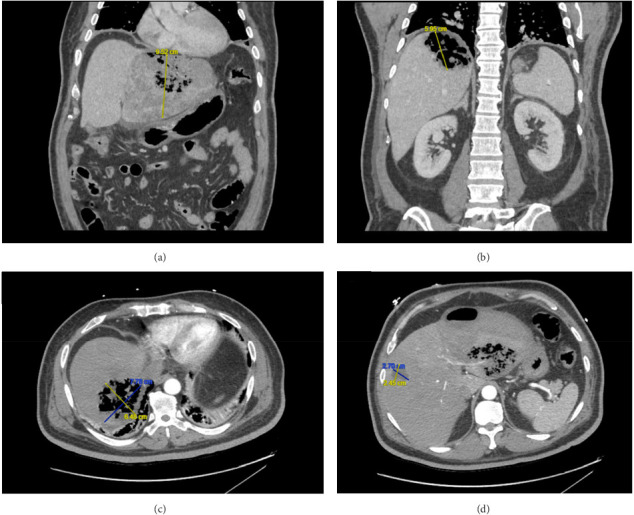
CT of the abdomen with IV contrast. (a) Coronal view of gas occupying the left hepatic lobe measuring 14.4 × 10.2 × 9.5 cm. (b) Coronal view of an additional similar collection within the right hepatic lobe measuring 7.8 × 6.5 × 5.95 cm. (c) Axial view of an additional similar collection within the right hepatic lobe measuring 7.8 × 6.5 × 5.95 cm. (d) Axial view of rim-enhancing collection in the right hepatic lobe measuring 2.8 × 2.7 × 2.45 cm.
